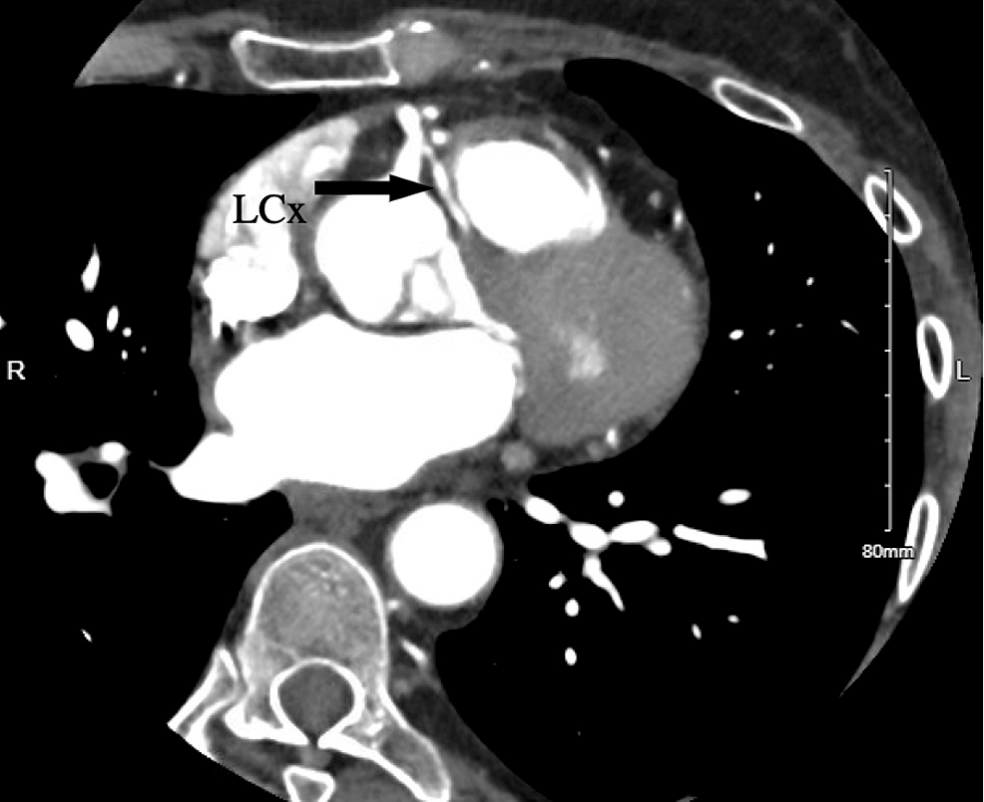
Coronary computed tomography angiography (CCTA) image showing left circumflex artery (LCx) arising from the right sinus of Valsalva (RSV) taking the malignant interarterial course between the pulmonary trunk and ascending aorta
